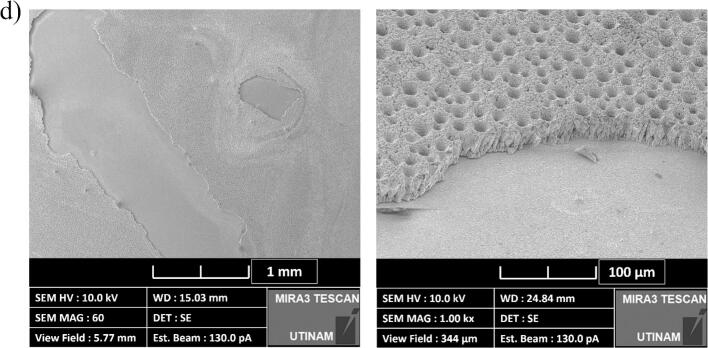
SEM images of the DHBT electrode erosion at various ultrasonic conditions after 30 min of exposure (a) 580 kHz – 14.5 W, (b) 20 kHz – 67.0 W, (c) 20 kHz – 32.8 W, and (d) 20 kHz PN – 20 W (Zoom: Left x60; Right x2,000 at 55°).
